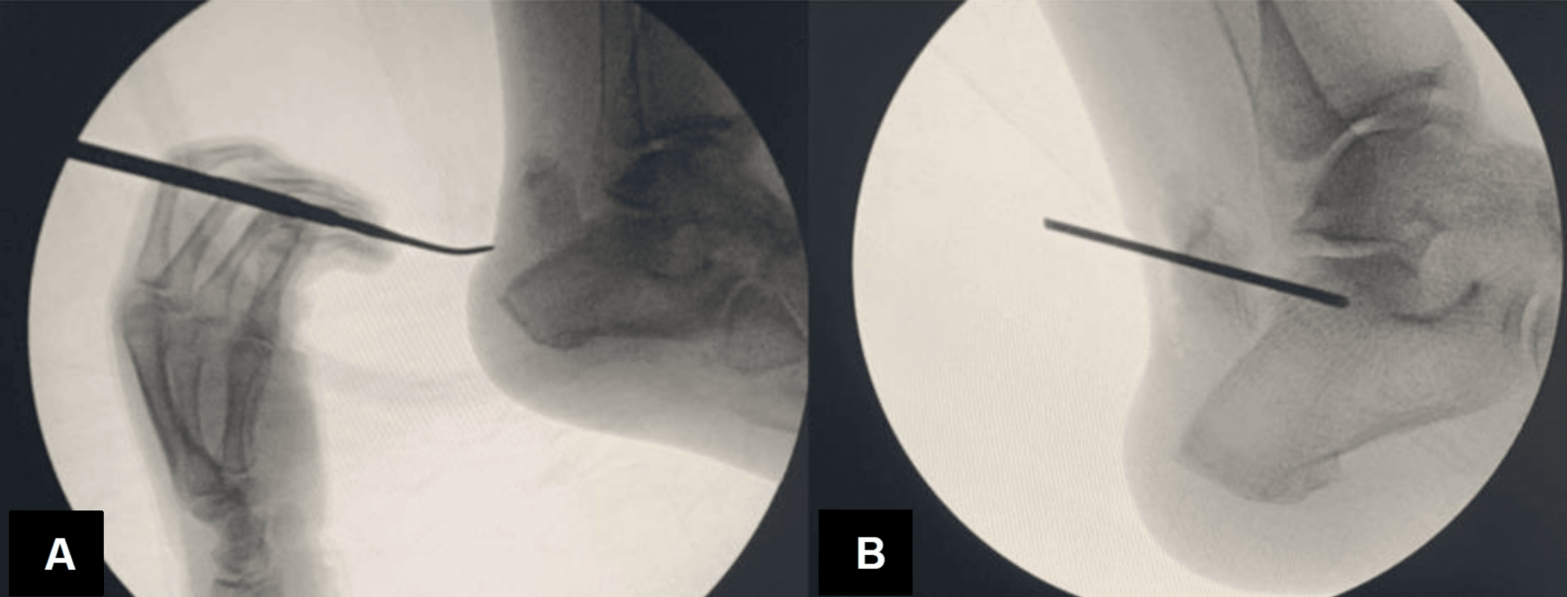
Fluoroscopic radiograph at the level of the calcaneal fracture fragment. Lateral intraoperative radiographs of the right foot immediately before and after initial calcaneal fracture pinning (A and B).
